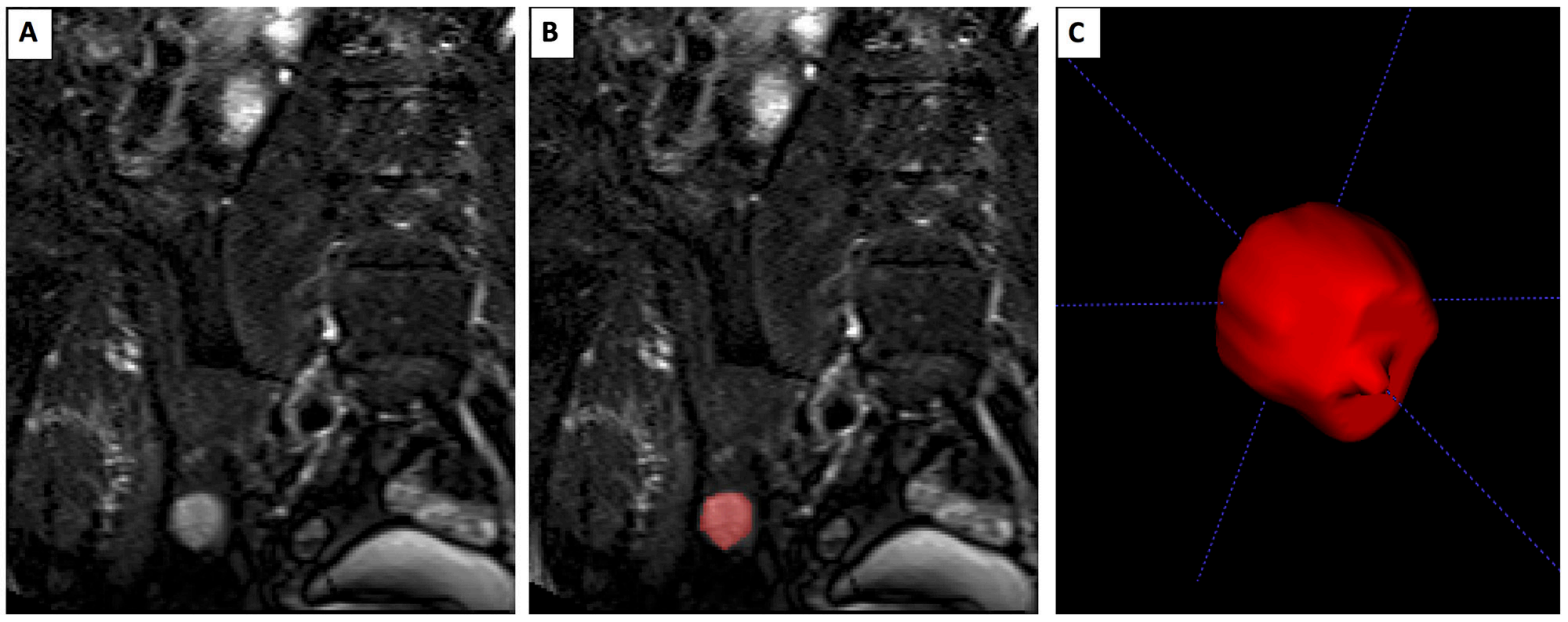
Segmentation of a focal lesion Extract of a T2-weighted coronal MRI sequence of abdomen/pelvis. (A) Native imaging with hyperintense focal lesion of the iliac bone in ITKSnap, (B) Image with focal lesion highlighted in red after 3D-segmentation was performed. (C) 3D-view of segmented focal lesion in ITK-SNAP.
